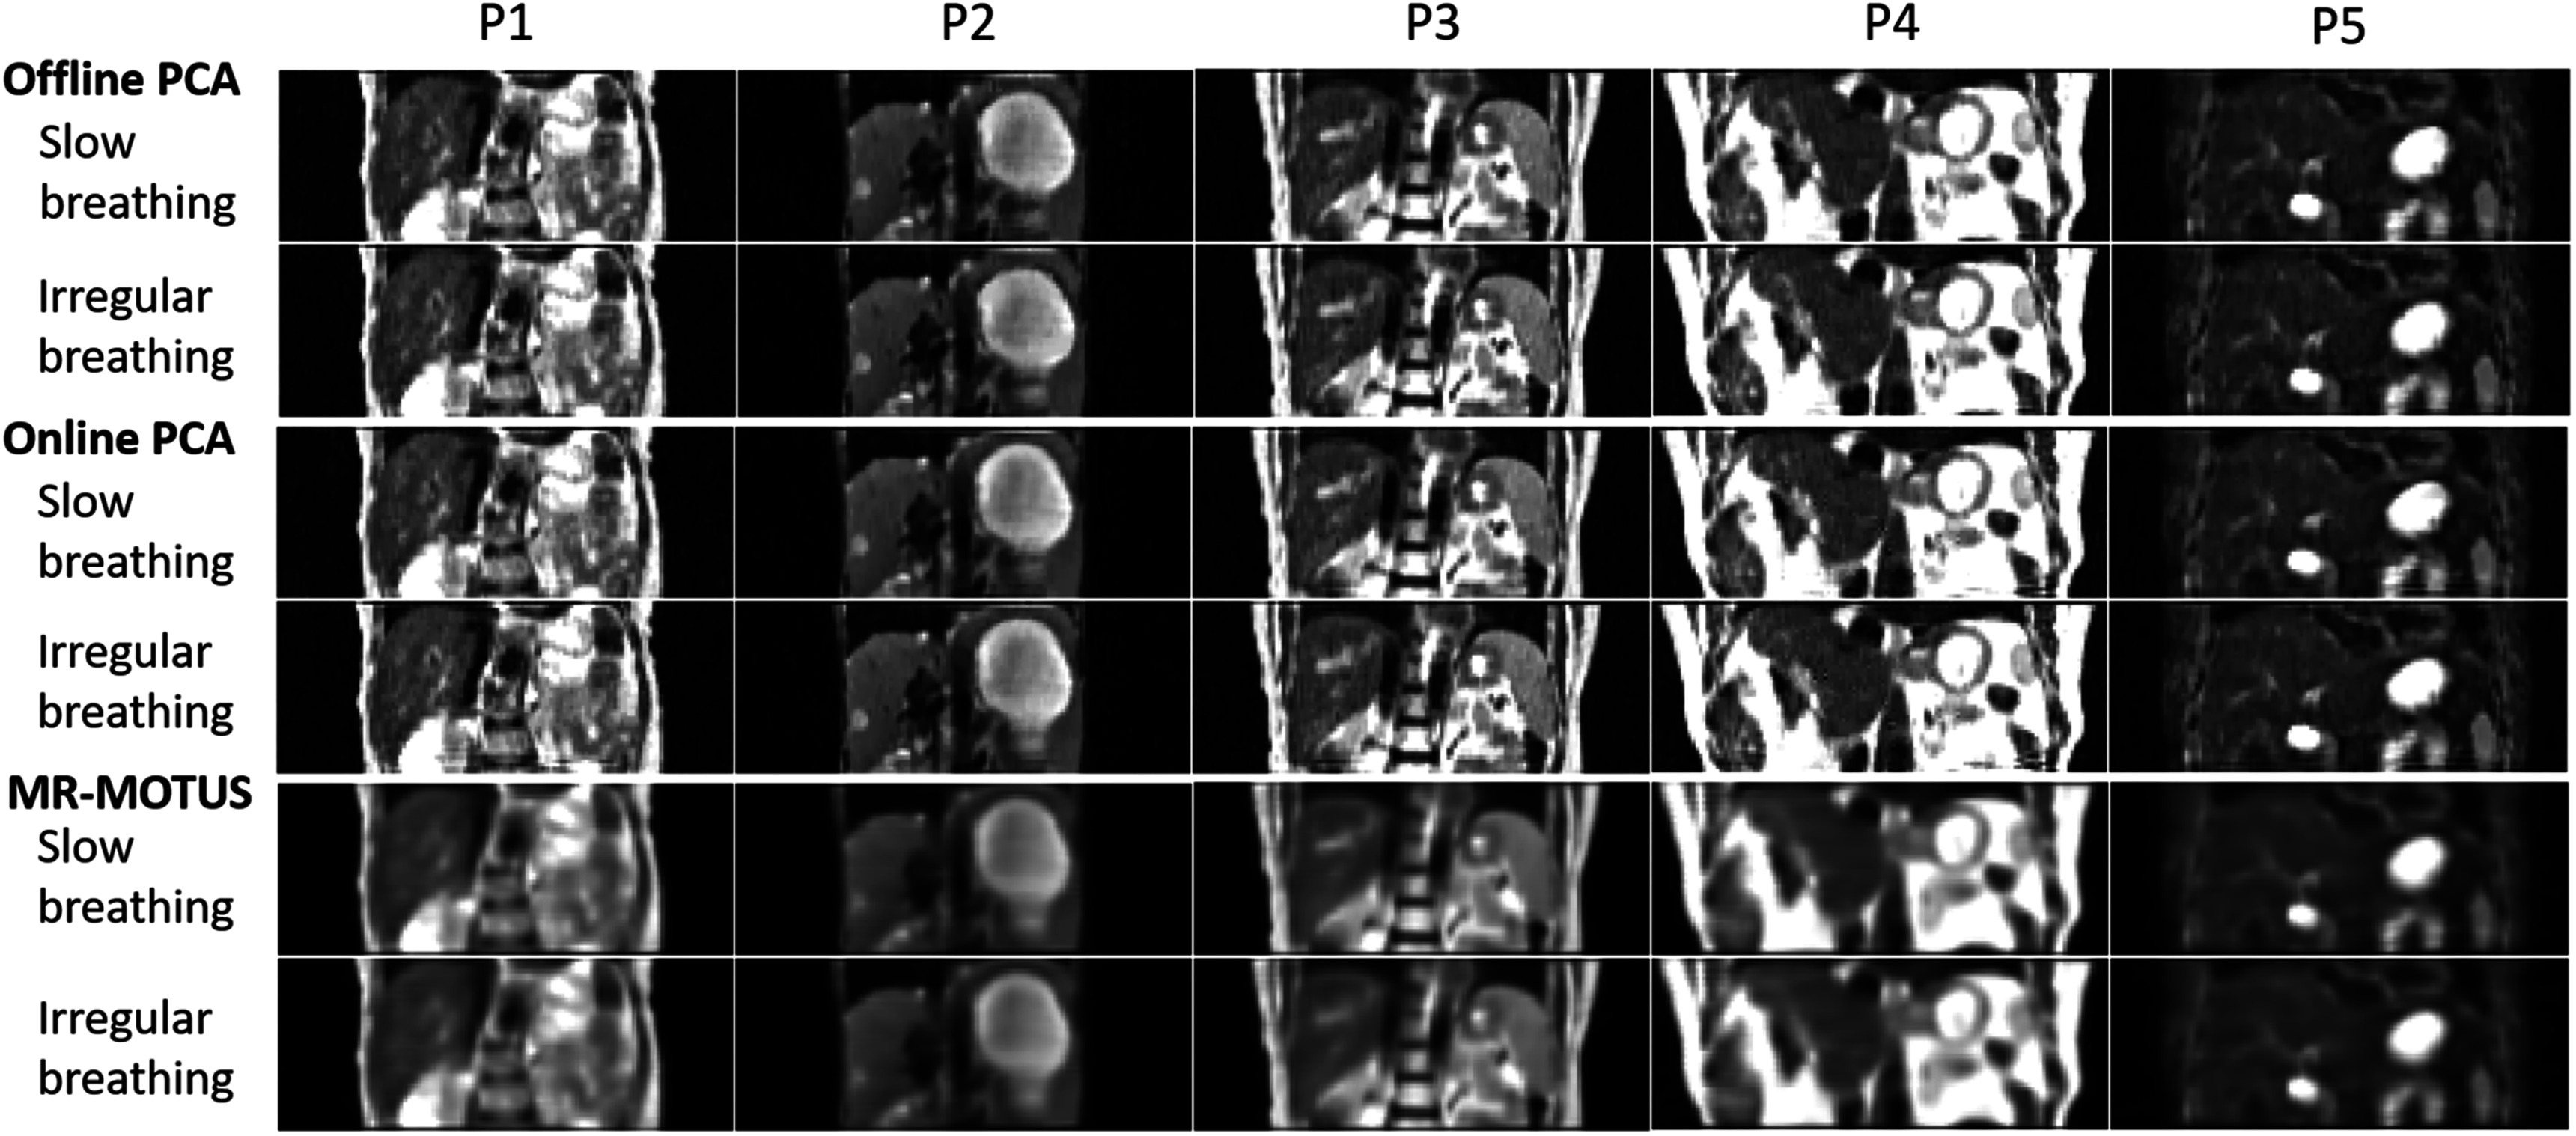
Comparison of reconstructed reference-frame MR images by STINR-MR (offline and online PCA) and MR-MOTUS for the slow and irregular breathing scenarios of the five patients (P1–P5) in the UTSW dataset.
